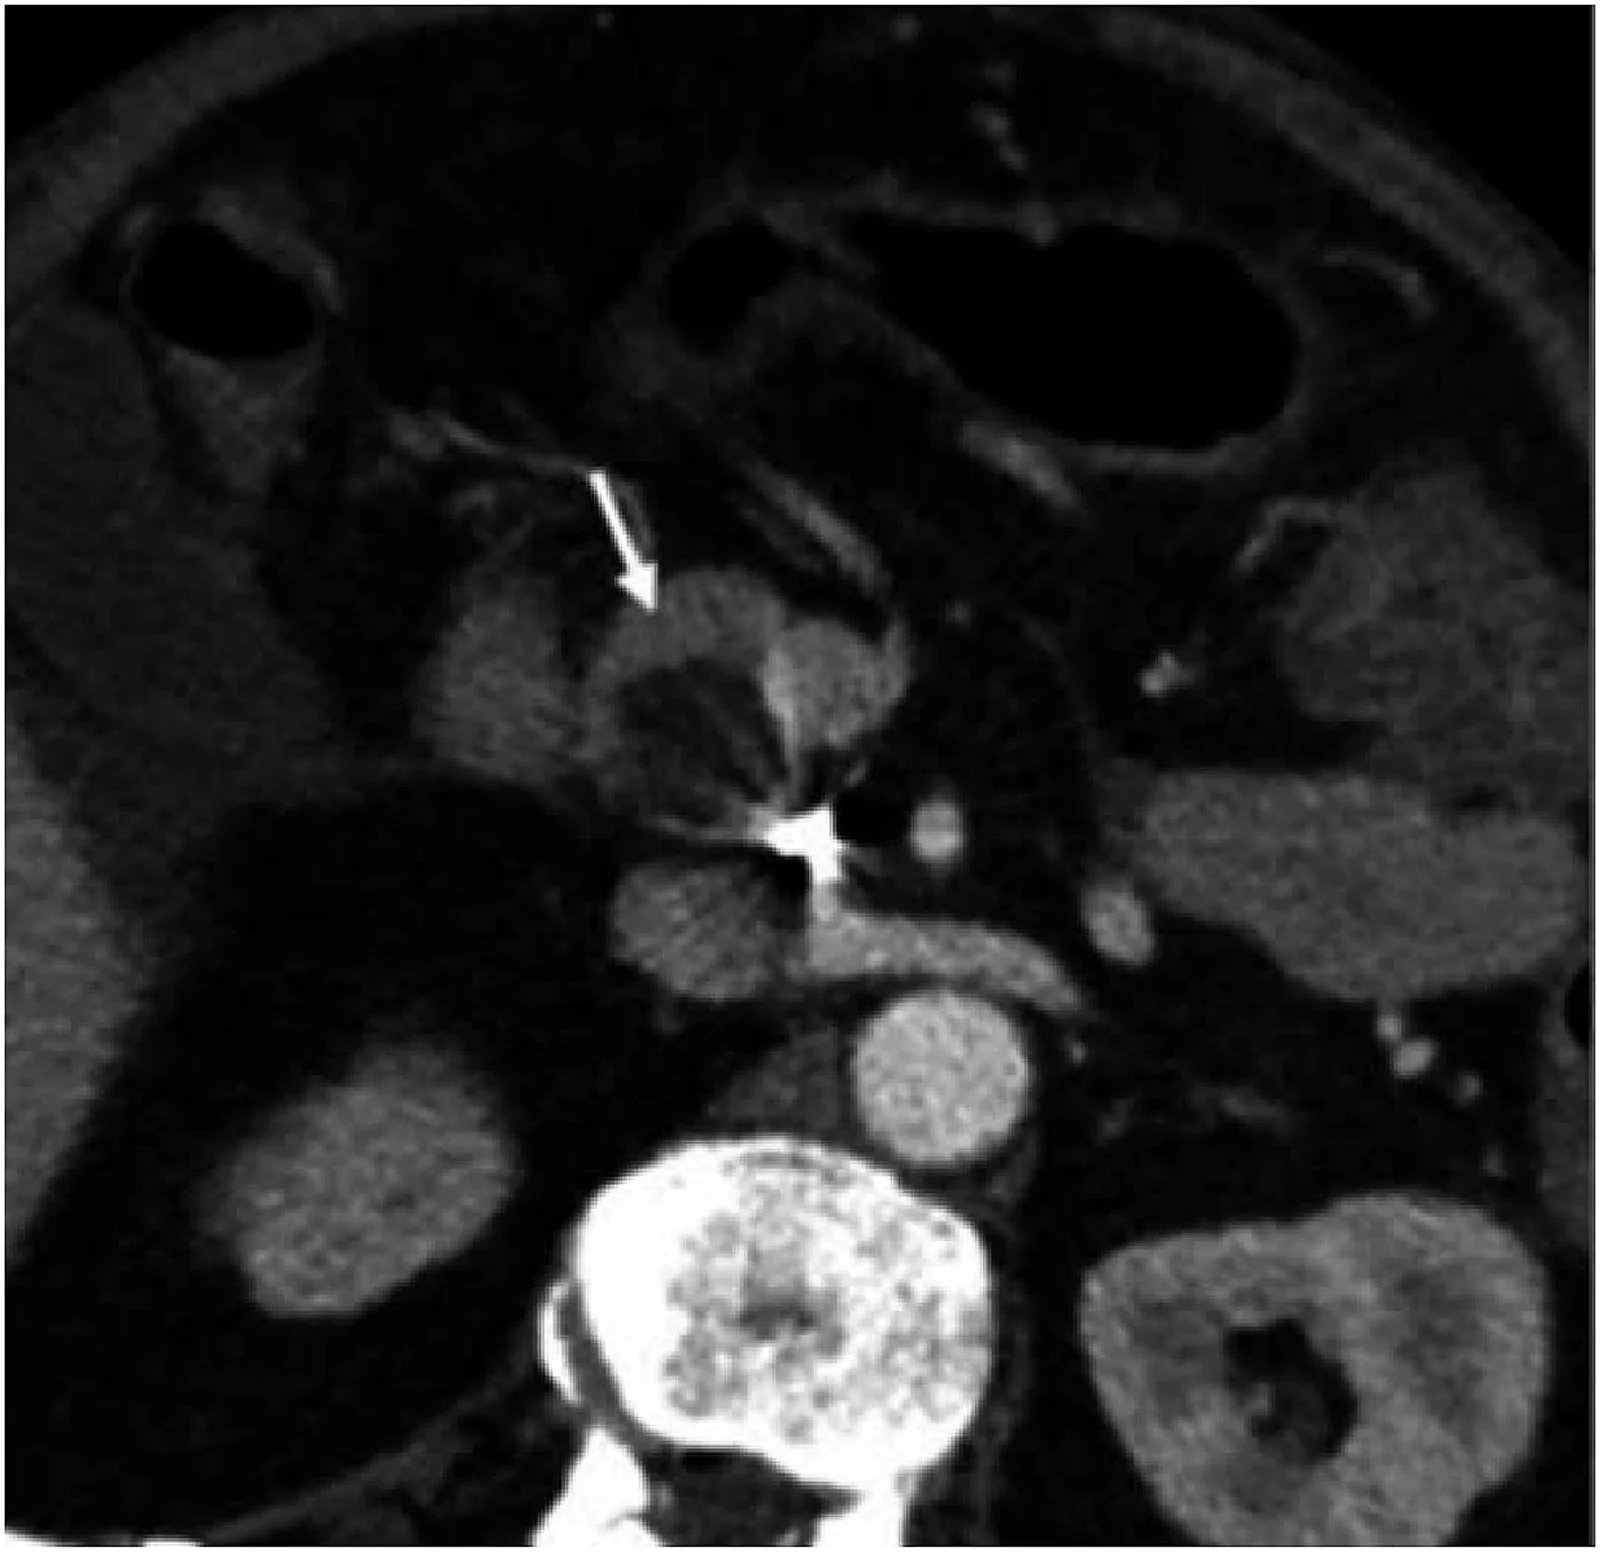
Follow up contrast enhanced CT scan showing complete thrombosis of the PSA.
